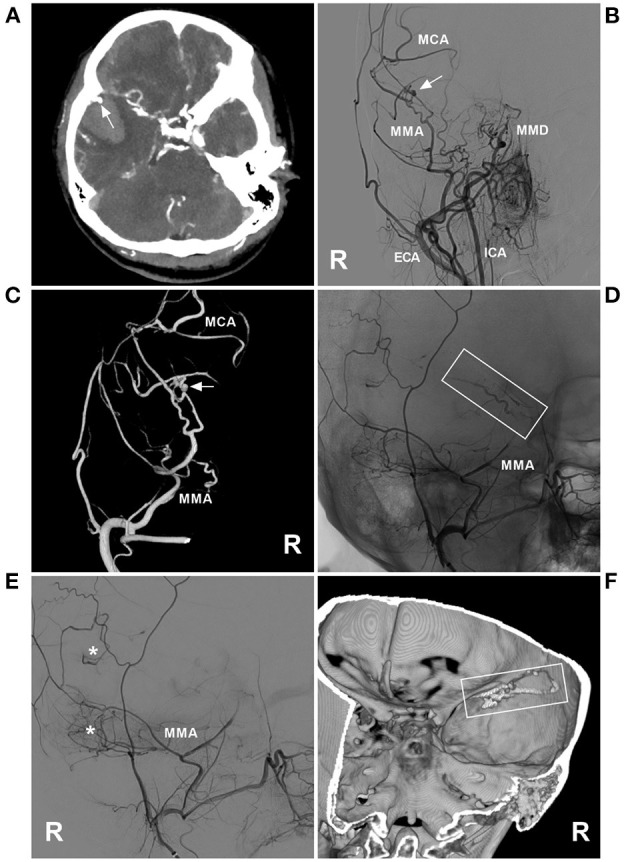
PAO of the MMA aneurysm in case 11 in Table 1. (A) Enhanced CT showing hemorrhage of the temporal lobe. An aneurysm (arrow) is indicated at the rim of the hematoma. (B) DSA of the right carotid artery showing MMD in the ICA; an aneurysm (arrow) was at the MMA. (C) Three-dimensional DSA of the right ECA showing an aneurysm (arrow) at the transdural location of the MMA; then, the MCA was continued. (D) Unsubtracted DSA showing the MMA as the parent artery to the aneurysm was occluded with Onyx (frame). (E) DSA of the right ECA showing that the petrous branch of MMD had anastomoses (asterisks) with the MCA. (F) Xper-CT showing the location of Onyx casting (frame). CT, computed tomography; DSA, digital subtracted angiography; ECA, external carotid artery; ICA, internal carotid artery; MCA, middle cerebral artery; MMA, middle meningeal artery; MMD, moyamoya disease; R, right.
